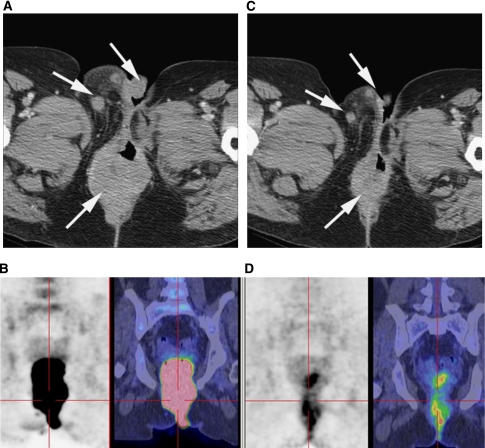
CT pelvis and FDG PET/CT images at baseline (A, B) and at 1 month (C, D) after treatment of a metastatic vulval melanoma with imatinib. Arrows indicate areas of response (Case 2).
